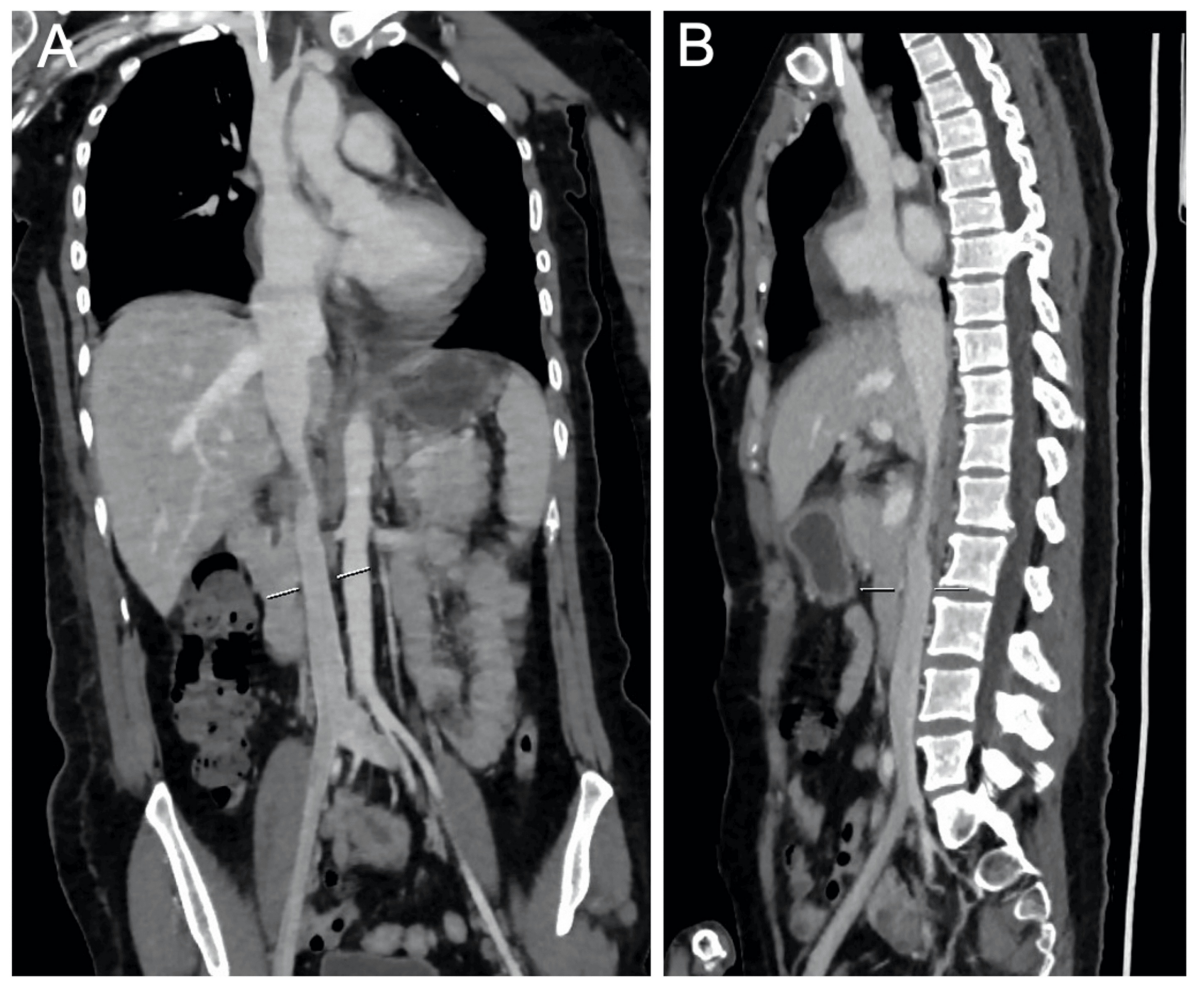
The seven-day post-operative CT scan shows the total extraction of the tumor. (A) The coronal view of post-operative CT scan shows total extraction of the tumor. (B) The sagittal view of post-operative CT shows no residual tumors.
